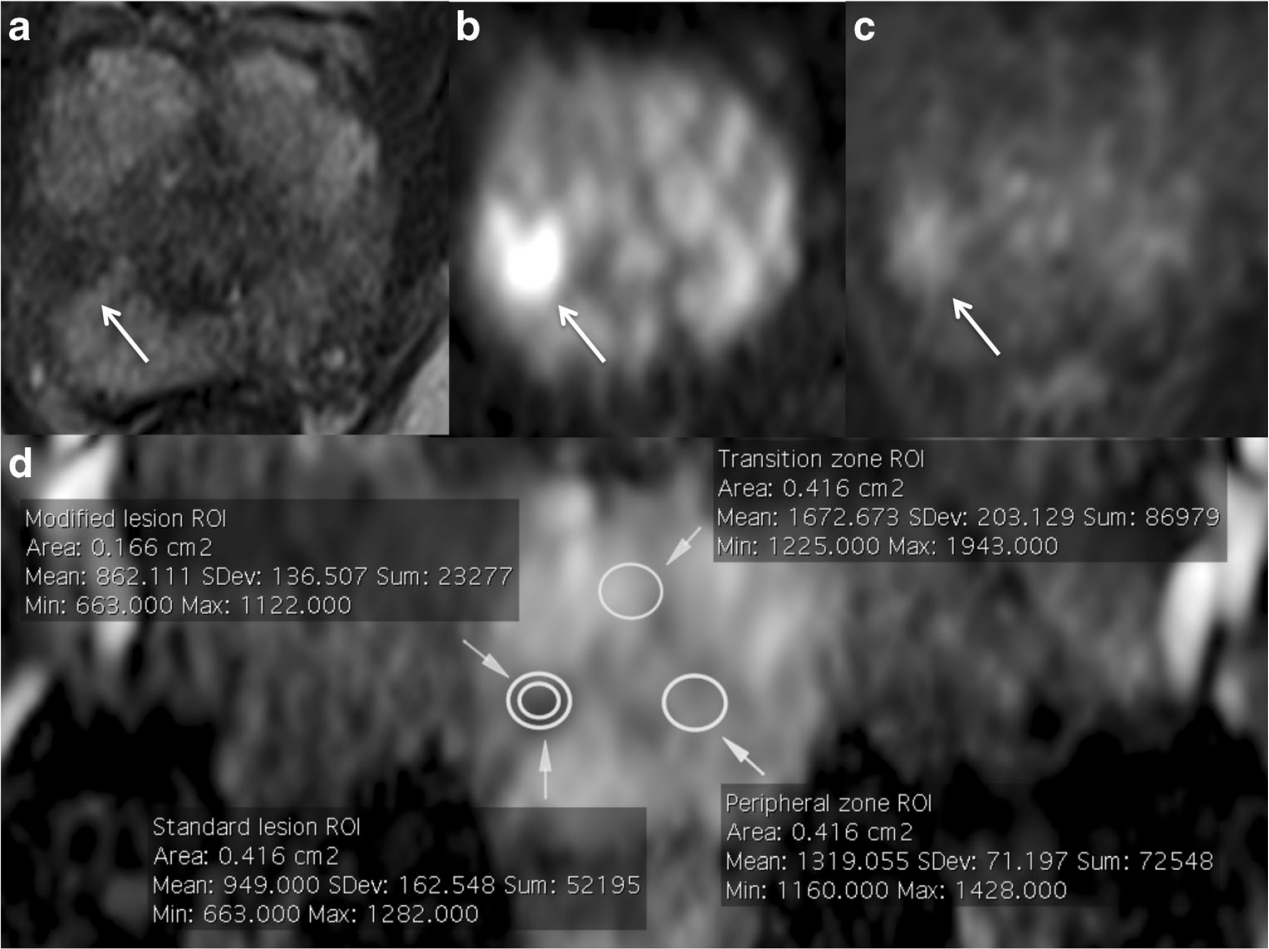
The arrows show a tumour in the right mid-apex peripheral zone of the prostate on T2-weighted (a), diffusion-weighted (b) and dynamic contrast-enhanced (c) imaging, and how all regions of interest (ROIs) were positioned on the same slice of the apparent diffusion coefficient (ADC) map (d) accordingly
